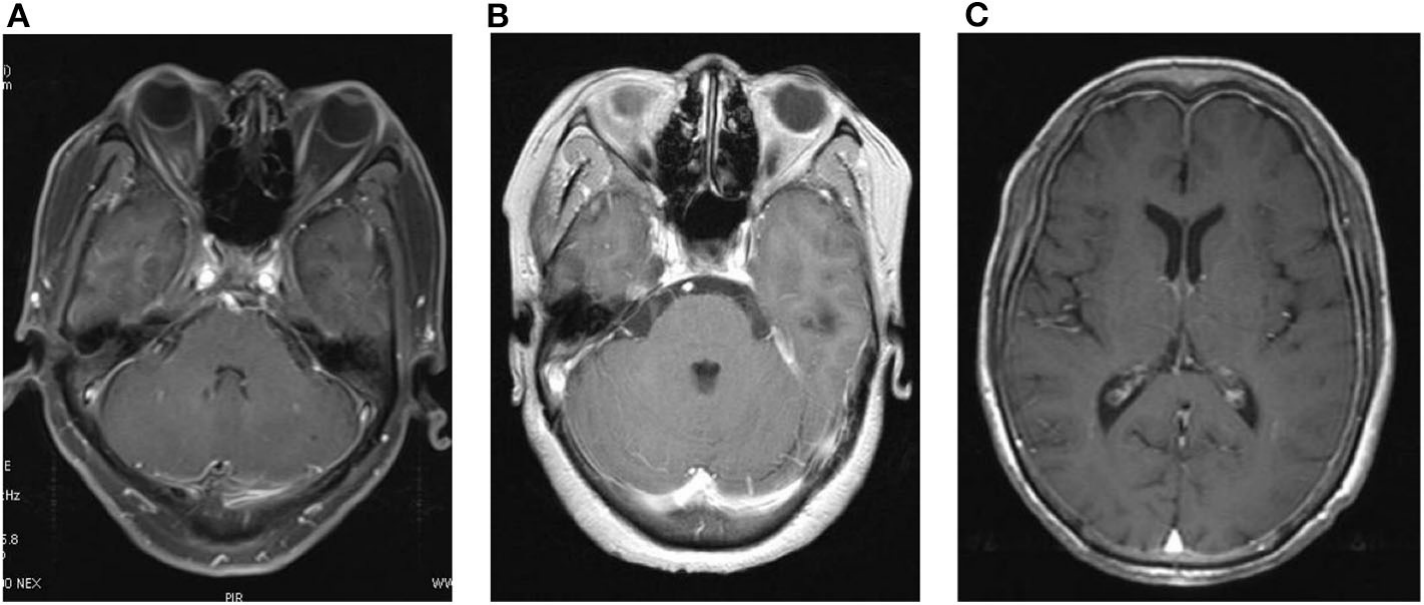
Typical radiology features for HP patients. (A) A 61 year-old man presented with headache and hearing loss. He was diagnosed with idiopathic HP. There was dural enhancement of the posterior fossa in an axial T1-weighted MRI. (B) Axial T1-weighted MRI of a 54 year-old woman demonstrated dural enhancement of the posterior fossa. She was diagnosed with tuberculous-related HP. (C) A 66 year-old woman complained of headache, right hearing loss, and right vision loss for 2 months. MPO antibodies were positive, and a diagnosis of ANCA-related HP was established. The enhanced MRI showed dural enhancement of the frontal, parietal, and occipital lobes.
